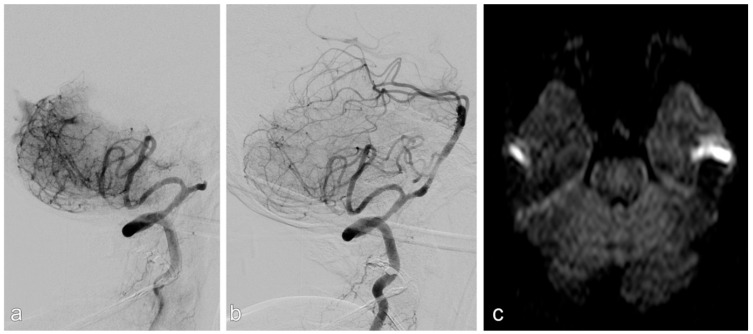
Patient with acute stroke due to acute occlusion of the basilar artery (a). After mechanical thrombectomy, there was a high-grade stenosis, which was stented successfully (b). There was no infarction on MRI upon discharge (c).
